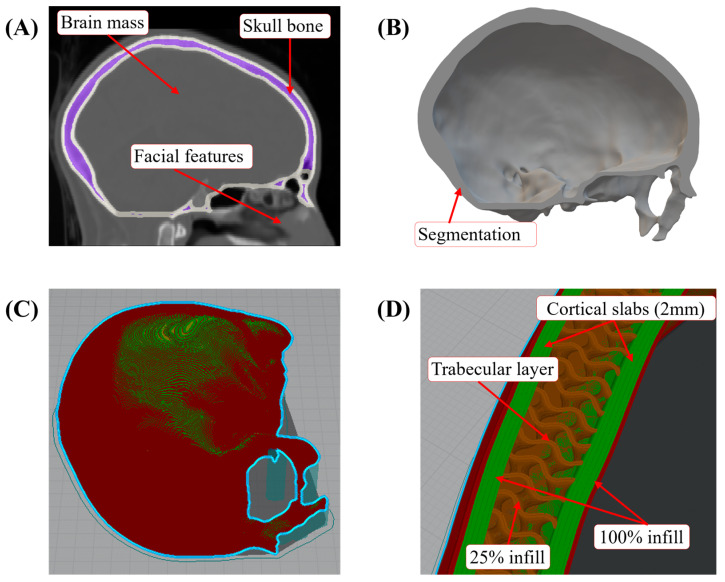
(A) Reference CT scan. (B) Segmented CAD model with only the skull of the cranial vault. (C) Layout of half-skull for 3D printing. (D) Geometry and attributes of the trilayer structure.
